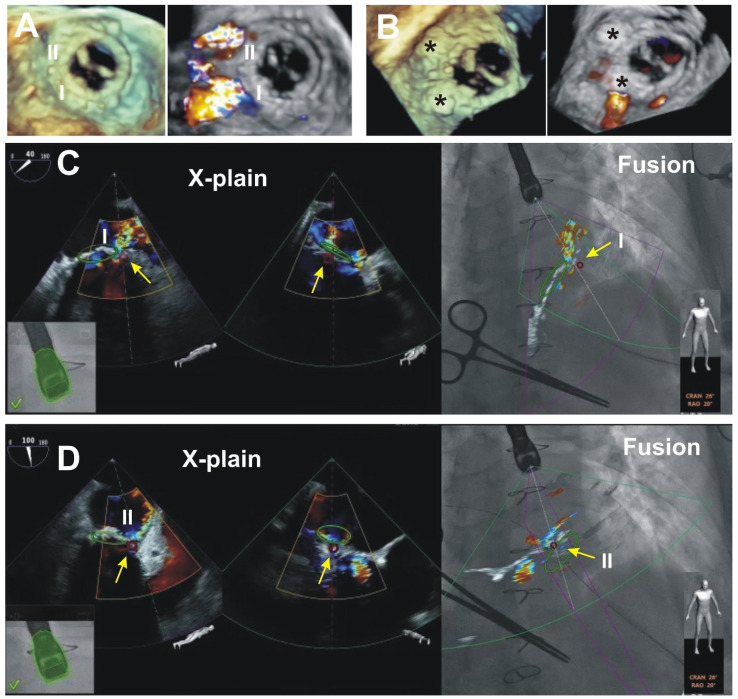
An example of EFF scenario A “Side view of the TEE probe”. The position of the C-arm at 26° Cran, and 20° RAO, TEE probe is seen obliquely—from the side and the top. The automatic recognition of the TEE probe by EchoNavigator^®^-system software is highlighted by the green contouring. (A,B) preprocedural and postprocedural 3D and 3D Color Doppler TEE images of the biological prosthesis from the surgical perspective. I and II—paravalvular regurgitant jets show the location of two PVLs at 8 and 10 o’clock (A). Black stars mark PVL occluders with a small residual regurgitant jet near the first occluder (B). (C,D) intraprocedural EFF images for the closure of the I and II PVLs. Two X-plain (perpendicular to each other) 2D TEE views with Color Doppler cross the I and the II PVLs and regurgitant jets on the left. 2D TEE views planes seen on EFF images tilted in space. In addition, the X-plain views used to construct the markers transferred onto the EFF images (on the right). Green circle—sewing ring of X-ray negative biological valve prosthesis, small red ring (yellow arrows) placed at the PVLs.
